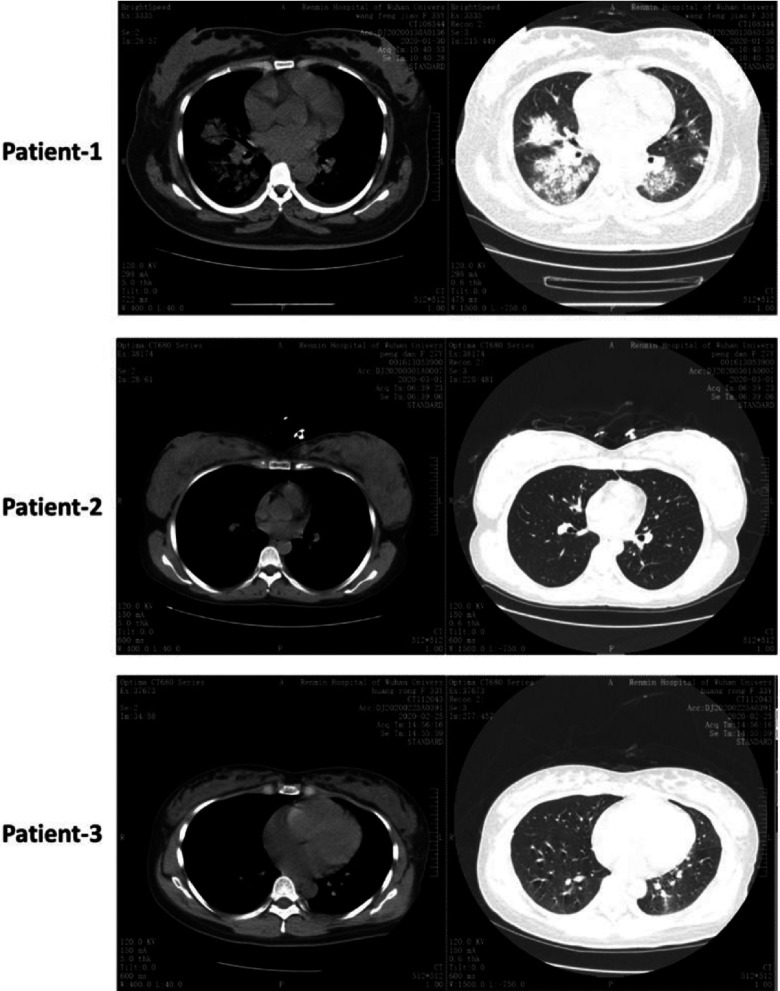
Computed tomography (CT) chest scans of 3 patients. This figure shows patchy consolidation and opacities.
